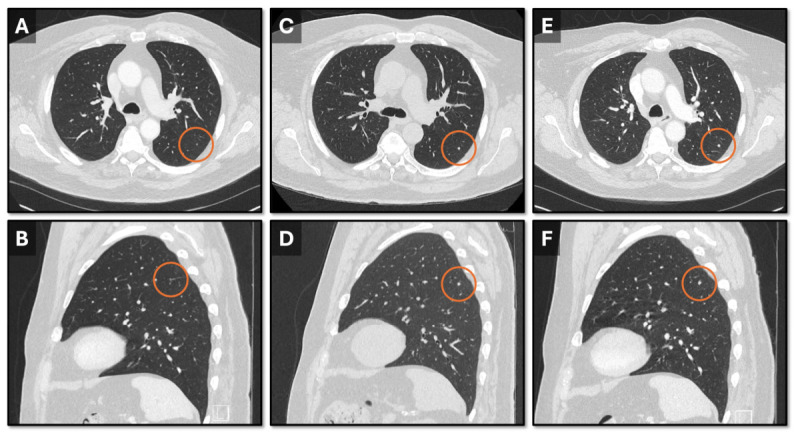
Left perifissural, biopsy-proven renal cell carcinoma (RCC). CT images at baseline in (A) axial and (B) coronal, 17 months from baseline in (C) axial and (D) coronal, and 23 months from baseline in (E) axial and (F) coronal. Nodule measures 2 mm at baseline, 4 mm at 17 months, and 6 mm at 23 months. The red circle highlights the concerning pulmonary nodule. Nodule was suspicious for malignancy based on interval growth and rounded morphology.
